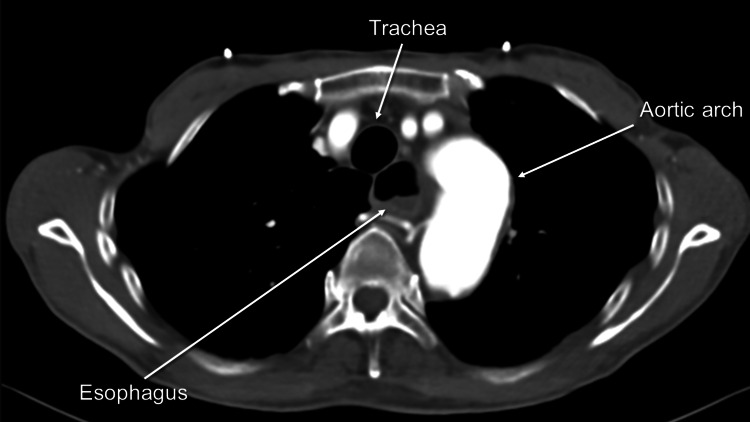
Contrast-enhanced computed tomography (CT) revealing dilated esophagus
